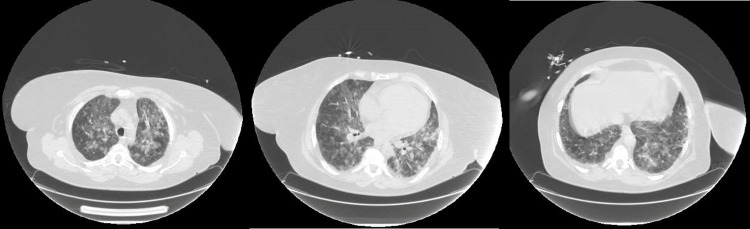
Chest CT scan, axial planes
